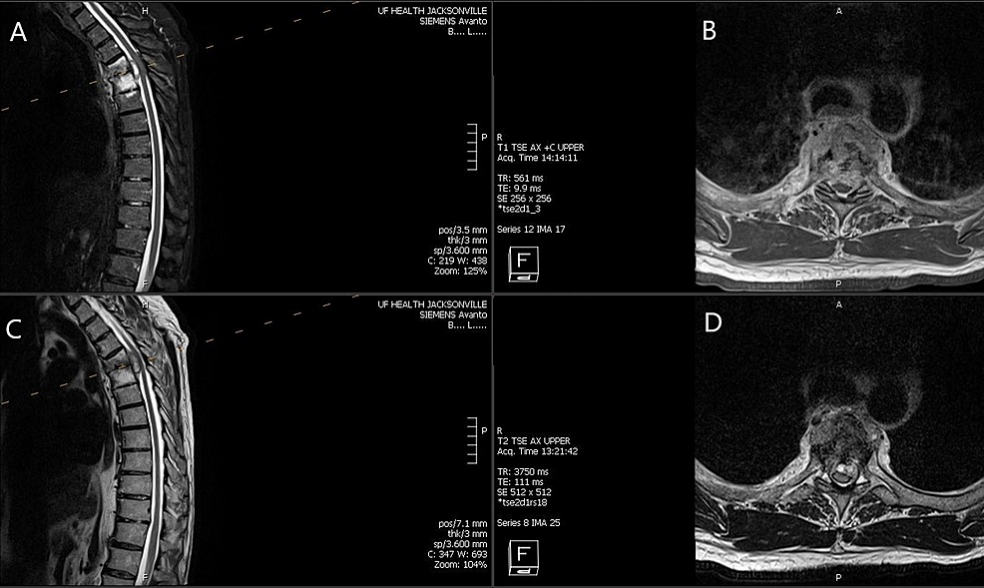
Preoperative MRI sagittal T2 STIR and T2 images (A, C), axial T1 with contrast and T2 (B, D) A: Sagittal T2 short tau inversion recovery (STIR) weighted images of thoracic spine revealing loss of disc space height at T4-T5 with increased T2 signal within the T4 and T5 vertebral bodies, erosion, and involvement of the disc space. There is a well-defined epidural mass lesion causing severe cord compression with cord changes. B: Axial T1-weighted MRI with contrast demonstrating enhancing lesion involving the disc space and adjacent vertebral body with cord compression. There is an abnormal enhancement of the T4 and T5 vertebral bodies with disc space involvement and pre-vertebral collection. C: Sagittal T2 image clearly showing the epidural mass as well as paraspinal involvement, erosion of the disc and the vertebral body with compression of the cord and the lesion which appears epidural. D: Axial T2 images revealing epidural involvement circumferentially more on the left side and ventrally located causing dural/cord compression with involvement of the prevertebral region and hyperintensity within the adjacent rib
